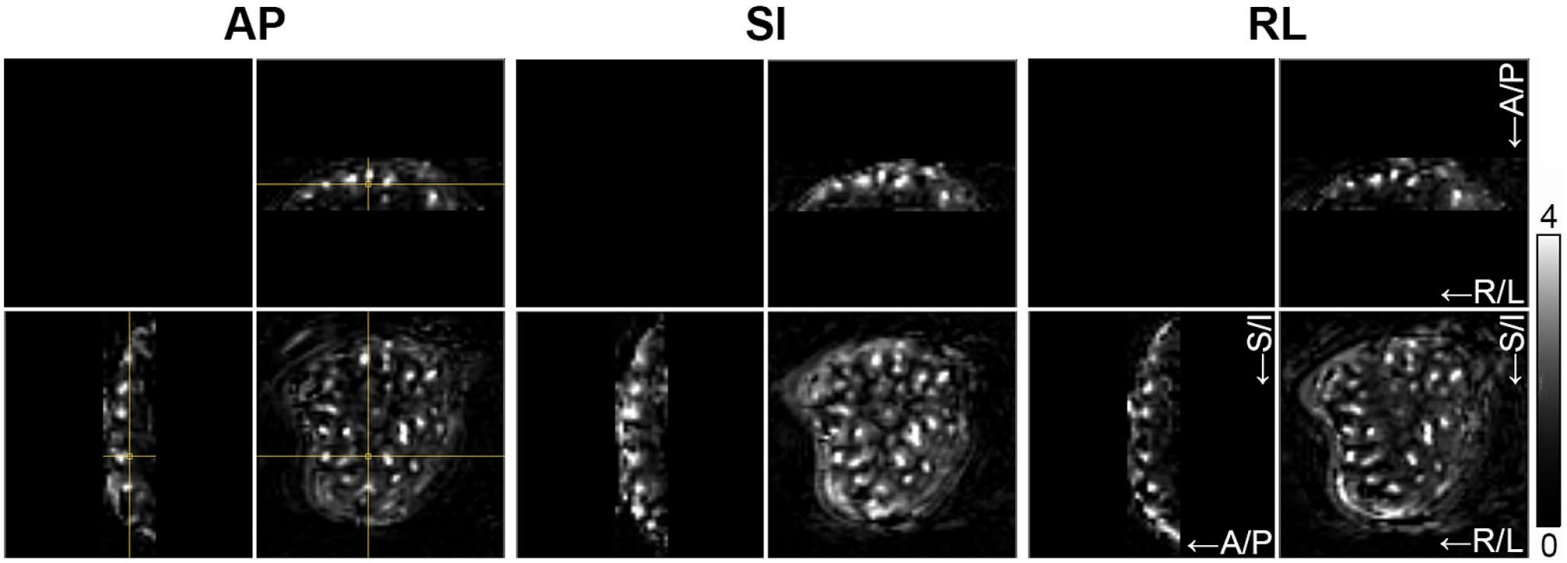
Variation of velocity encoding direction. Placental perfusion images obtained with VS‐ASL MRI using velocity encoding along each of three orthogonal directions (AP, SI, and RL) in one pregnant volunteer (Subject 1 in Table 1). Orthogonal slices (spatial resolution: 4 × 4 × 4 mm^3^) are shown. The cross‐hair in the left images indicates the intersection of the slices. Images have equal LUT scaling. The grayscale bar indicates PWS [%]. A/P, anterior‐posterior; R/L, right‐left; S/I, superior‐inferior
